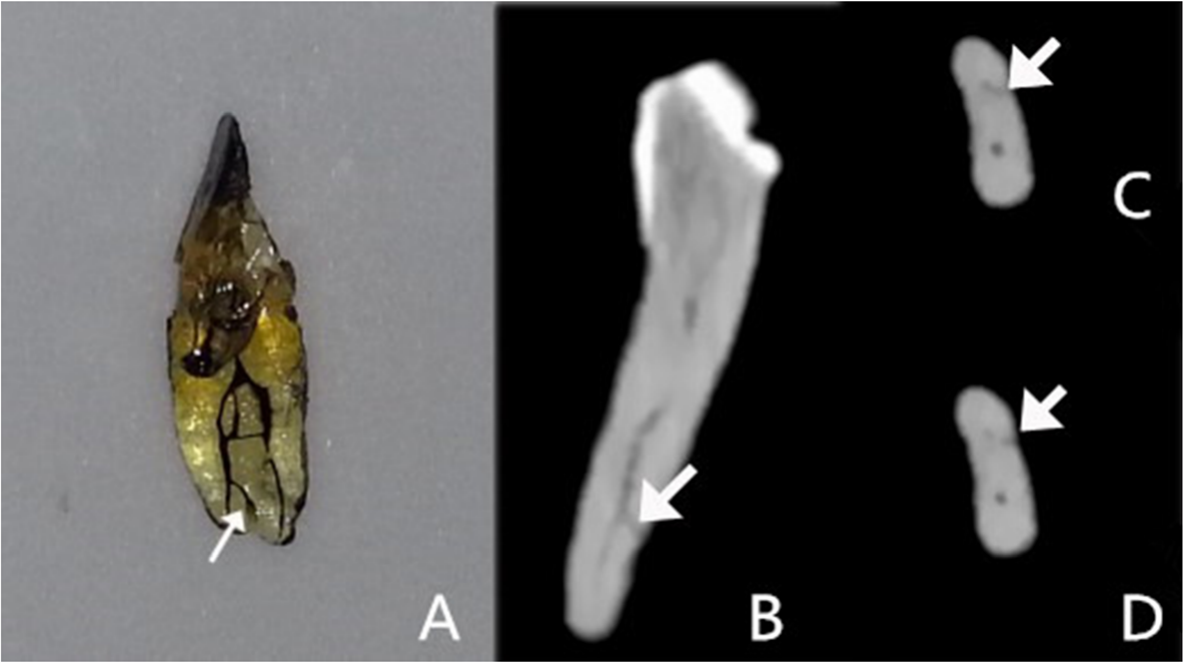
Image of a tooth with lateral canals. The white arrows denote the lateral canal. a Cleared tooth demonstrating the lateral canal. b CBCT image with oblique reconstruction. c and d CBCT images in the axial view: A subtle branch can be seen extending from the labial canal to the root surface
